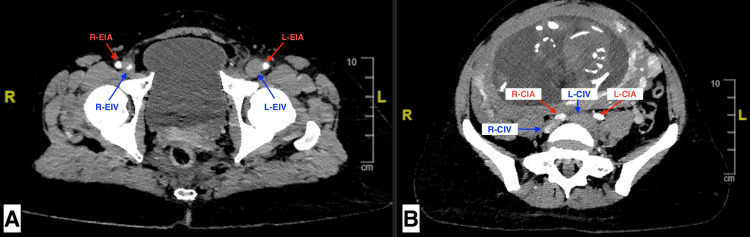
CT venography showing thrombus extension from the left external iliac vein to the left common iliac vein A. Filling defect and dilation of the left external iliac vein (L-EIV) compared to the right external iliac vein (R-EIV). B. Filling defect and dilation of the left common iliac vein (L-CIV) compared to the right common iliac vein (R-CIV), due to extrinsic compression by the right common iliac artery (R-CIA) and the gravid uterus. CT: computed tomography
